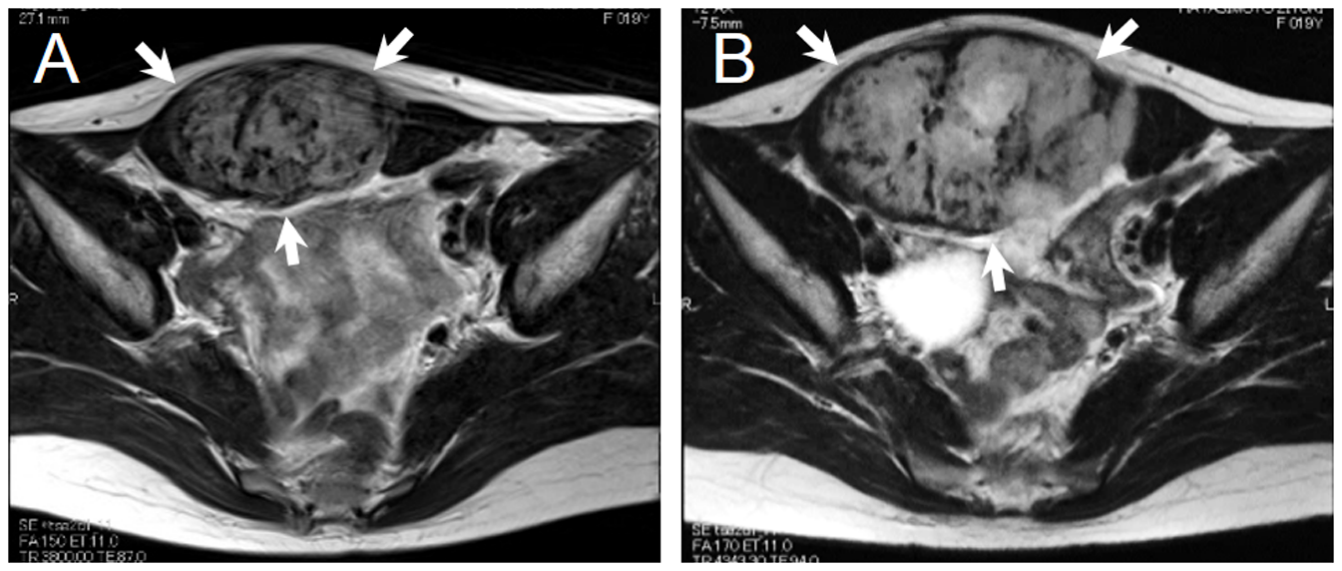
Twenty year-old woman with a desmoid tumor arising in the abdominal wall. Evaluation for efficacy of meloxicam according to RECIST criteria was PD. This tumor had S45F mutation. (A) A T2-weighed axial MRI of desmoid (arrows) at the first visit. (B) A T2-weighed axial MRI of desmoid (arrows) at the end of meloxicam treatment.
